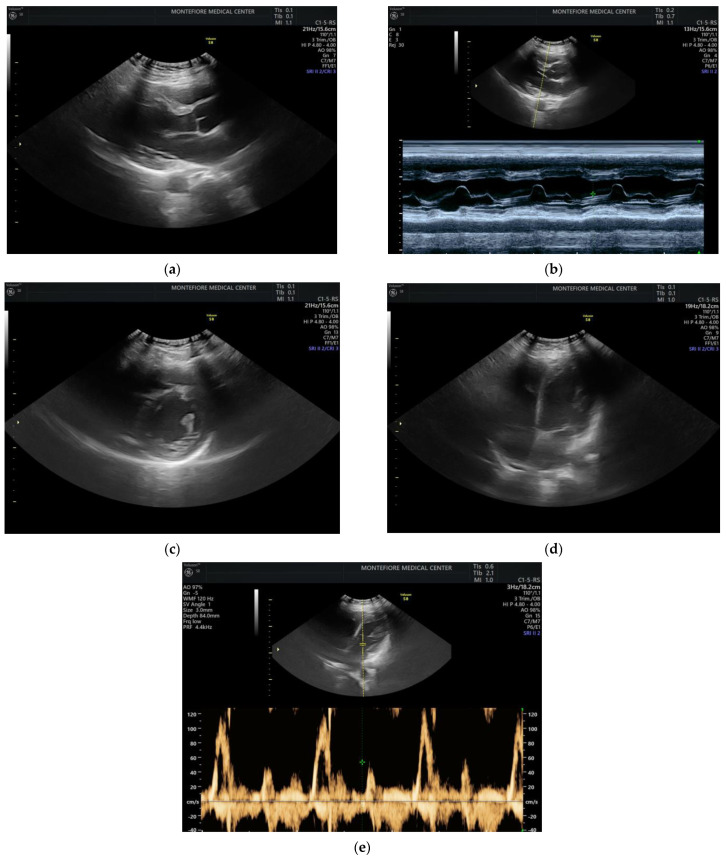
Performing cardiac POCUS on a pregnant patient using an OB probe may provide adequate diagnostic information. (a) Parasternal long axis view. (b) M-mode of the mitral valve. (c) Parasternal short axis view. (d) Apical 4-chamber view. (e) Pulse wave Doppler.
